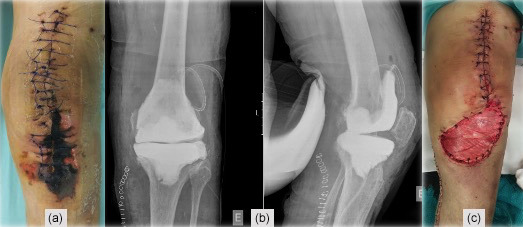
(a) Skin necrosis on the fifth day after DAIR, (b) X-ray after the implant
